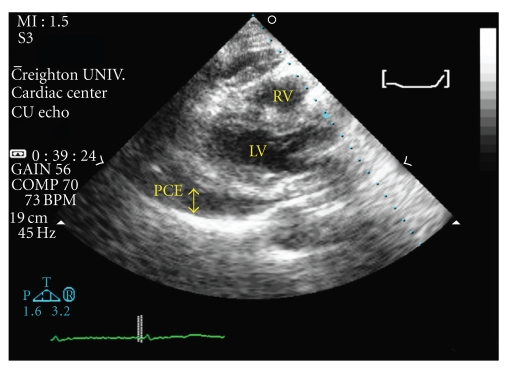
Transthoracic echocardiogram in parasternal long axis view showing the large pericardial effusion. LV: left ventricle; RV: right ventricle; PCE: pericardial effusion.
